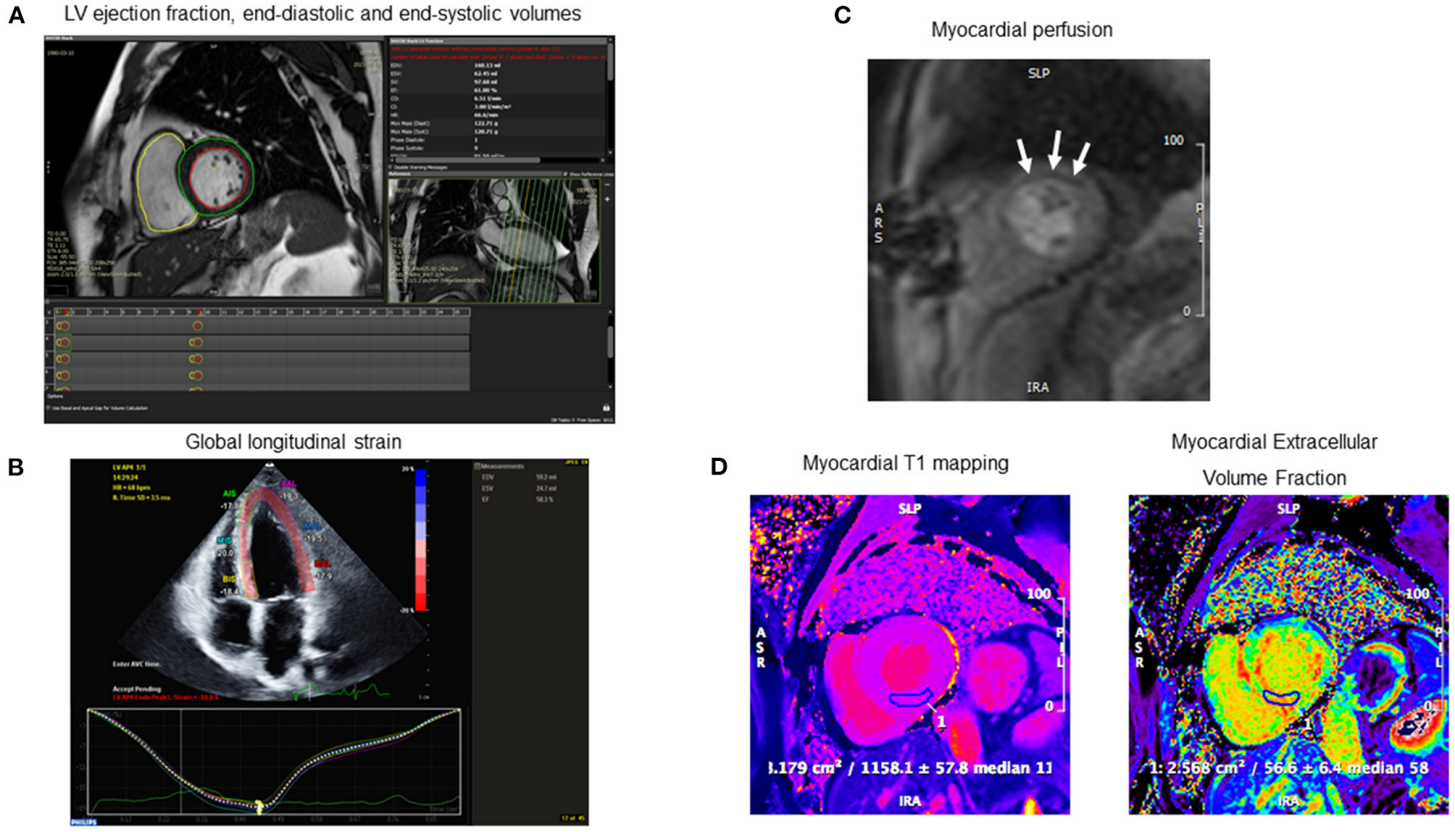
Examples of cardiac imaging metrics to assess the effects of exercise training in patients with or at risk for cardiovascular disease. (A) Left ventricular volumetric analysis of short-axis steady state free precession (SSFP) images acquired from cardiac MRI. (B) Global longitudinal strain acquired transthoracic echocardiogram. (C) Myocardial perfusion acquired from first-pass gadolinium enhanced imaging on vasodilator stress cardiac MRI. Note anterolateral perfusion defect (white arrows). (D) Myocardial tissue characterization on cardiac MRI with native T1 mapping (left) and extracellular volume fraction (ECV) (right). Note the elevated myocardial T1 (1,158 ms) and ECV (57%) consistent with cardiac amyloidosis.
